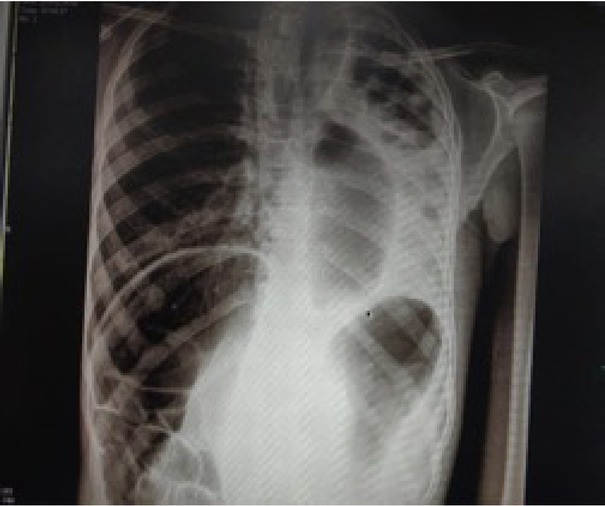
X-ray chest and abdomen of one patient showing complete collapse of the left lung, hyperinflation of the right lung and the Chilaiditi sign.
